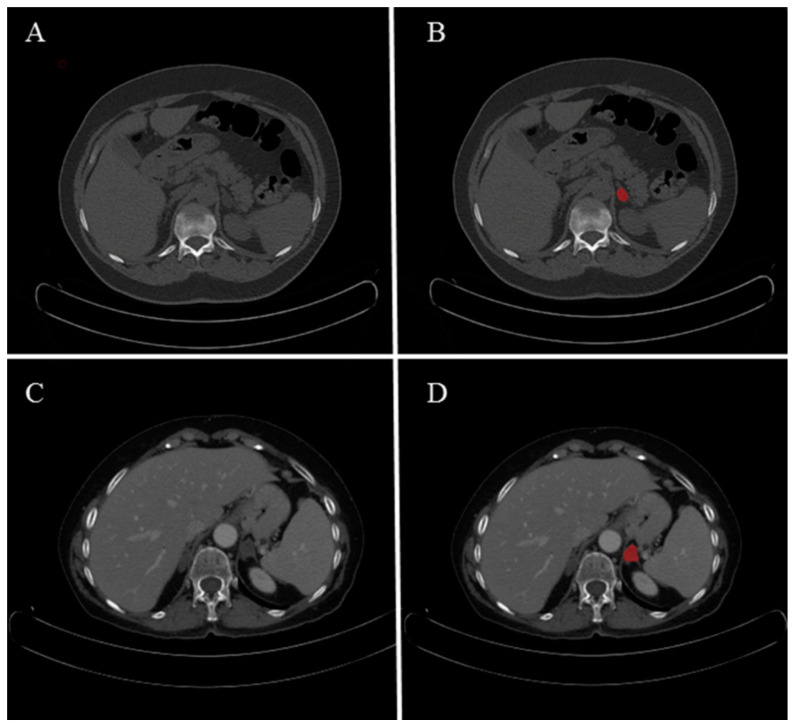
Segmentation example for unenhanced (A,B) and contrast-enhanced (C,D) CT. Images on axial plane with ROIs in red.
